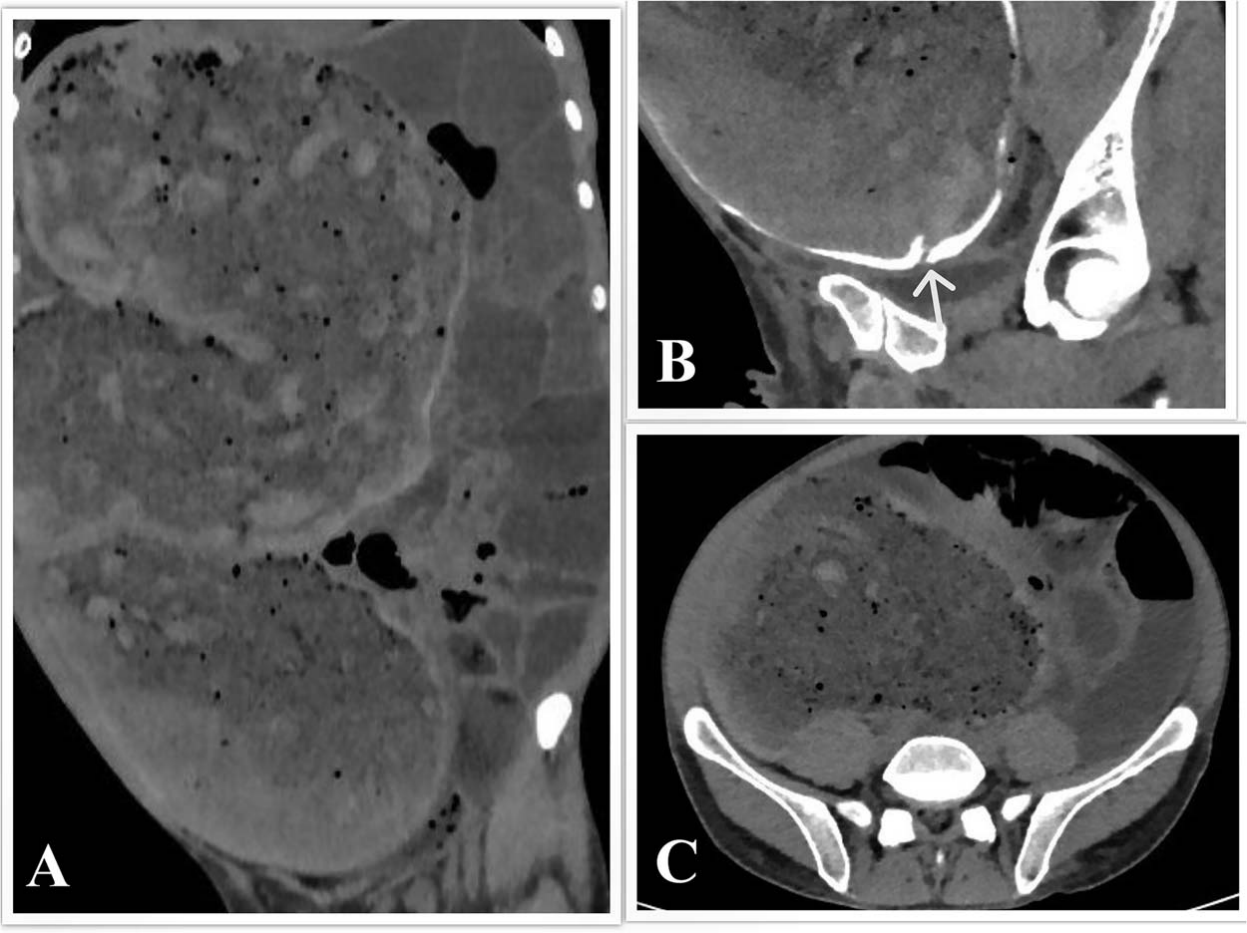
CT scan of the abdomen and pelvis. (A) Coronal view showing the megacolon and small bowel dilatation. (B) Sagittal view showing the sigmoid stricture, with the arrowhead pointing toward it. (C) Axial view showing colon dilatation at the mid-sigmoid level.
